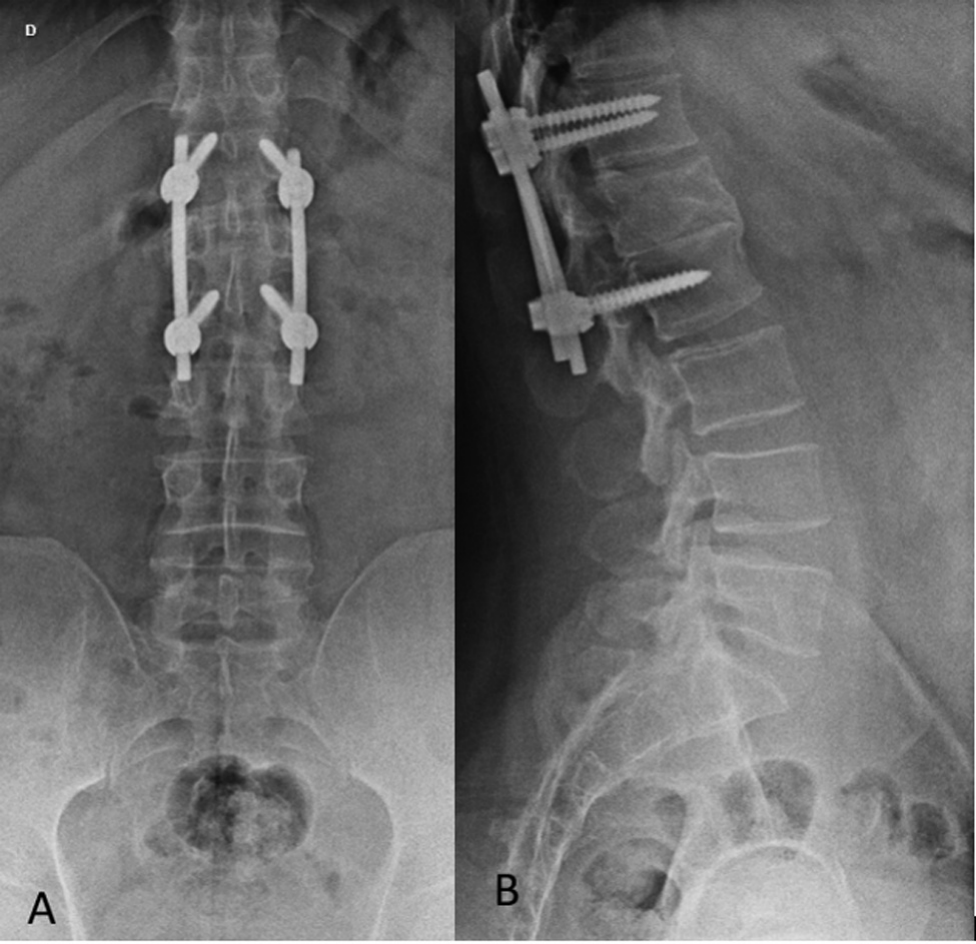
Immediate post-operative radiograph of a patient undergoing minimally invasive percutaneous fixation in anteroposterior (A) and lateral (P) views.
